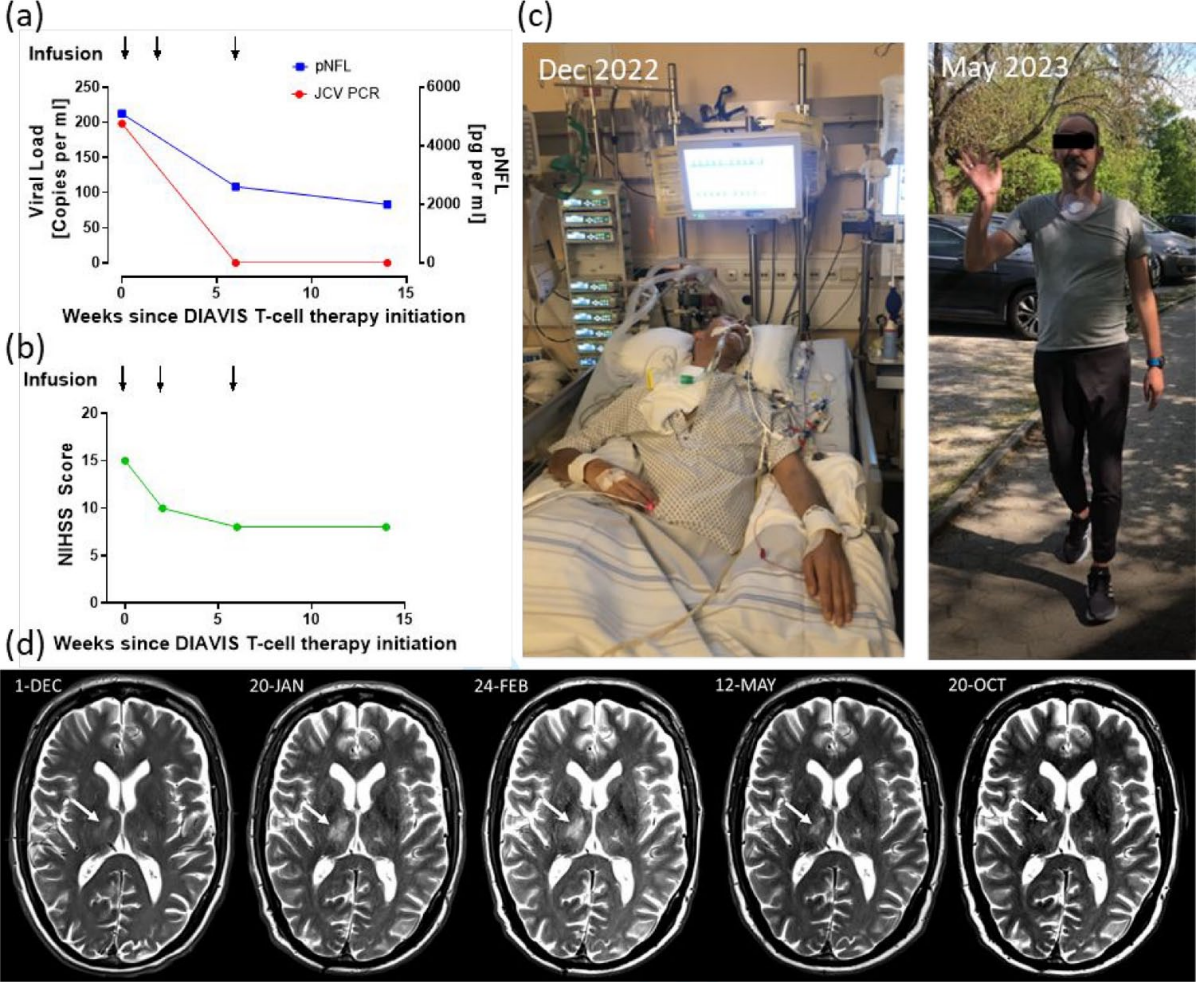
Clinical and laboratory follow-up parameters. (a) JCV viral load (red) and pNFL concentration (blue) on multiple time points since therapy. Black arrows: Times of DIAVIS T-cell infusion. (b) NIHSS score on multiple time points since therapy initiation. Black arrows: Times of DIAVIS T-cell infusion. The score was determined retrospectively on the basis of available neurological examination results. (c) These photographs show the severe clinical condition of the patient, with the left image taken before the initiation of DIAVIS BKV and EBV T-cell treatment, and the right image captured 19 weeks following the commencement of therapy. The photographs were taken by the patient’s relatives. We have obtained the patient’s consent to share these photographs. (d) T2*-weighted MRI image before, during, and after therapy initiation. Despite initiation of HAART, the MRI revealed PML progression with enlarging lesions prior to therapy initiation, consistent with the clinical deterioration. After initiation of therapy with DIAVIS BKV and EBV T-cells in mid-January 2023, the lesions showed a steady regression. DIAVIS T-cells, directly isolated allogeneic virus-specific T-cells; HAART, highly active antiretroviral therapy; JCV, human polyomavirus 2; MRI, magnetic resonance imaging; NIHSS, National Institutes of Health Stroke Scale; PML, progressive multifocal leukoencephalopathy; pNFL, plasma neurofilament light chain.
